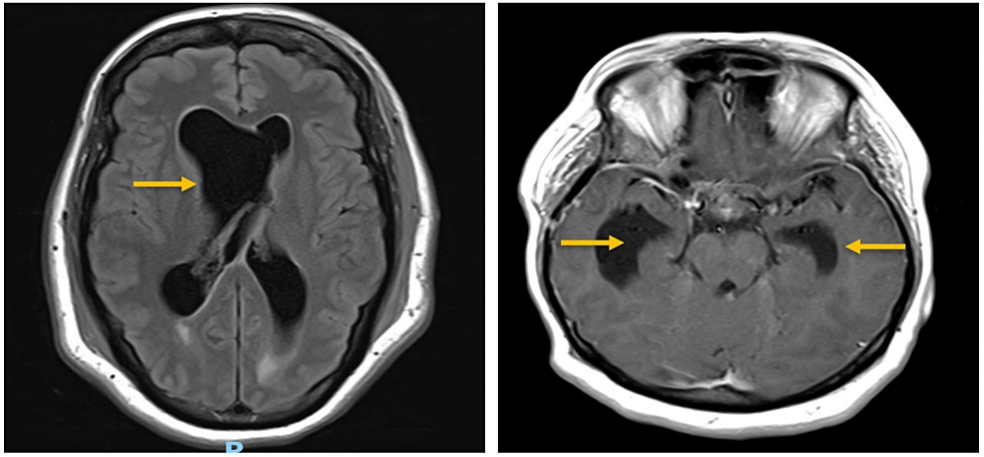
Axial MRI images showing communicating enhancing hydrocephalus (orange arrows)
